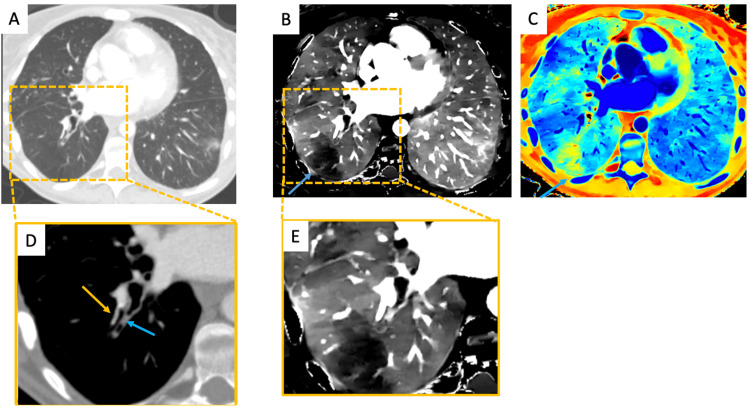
A 34-year-old male with a history of lung transplant presenting with acute right-sided chest pain with a D-dimer of 900 ng/mL. Initial axial CT scan of chest lung window setting (A) demonstrates no remarkable abnormalities. The corresponding iodine density map (B, E) and the Z_eff_ maps (C) show a peripheral wedge-shaped perfusion defect in the right lower lobe (blue arrow), mimicking pulmonary embolism. However, there is no evidence of pulmonary filling defect within the right lower lobe arterial branch supplying this region (tan arrow) on soft tissue window image (D) but a focal mucous plug within the segmental bronchus was detected (blue arrow), confirming that the perfusion defect is due to hypoxic pulmonary vasoconstriction. CT: Computed tomography
